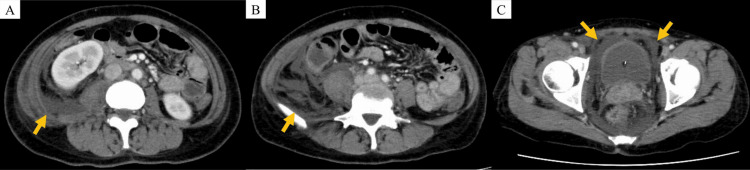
Contrast-enhanced CT findings on Day 3 of hospitalization (A, B) Increased fluid accumulation in the right retroperitoneal space. (C) Extension of fluid collection into the paravesical space.
